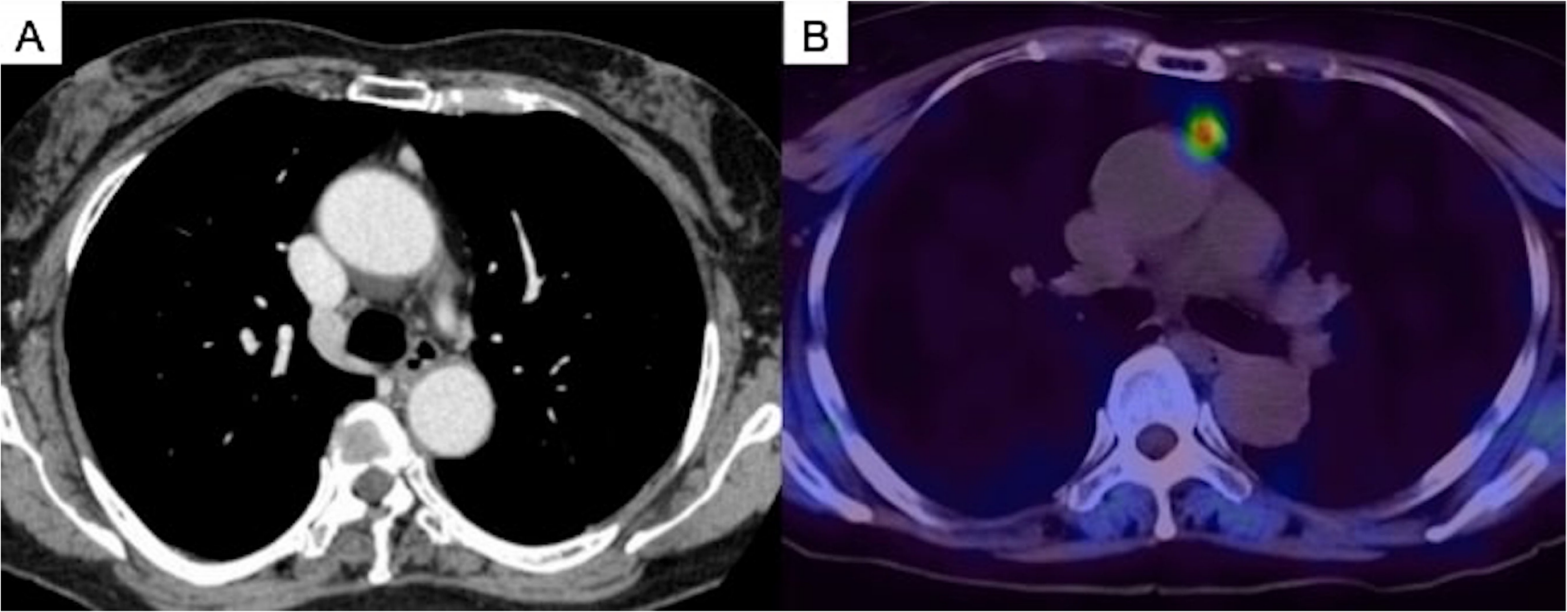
Imaging findings. (a) Chest computed tomography scan showing a 10-mm nodule in the anterior mediastinum. (b) Technetium-99 m methoxyisobutyl isonitrile scintigram showing an abnormal uptake lesion in the anterior mediastinum
